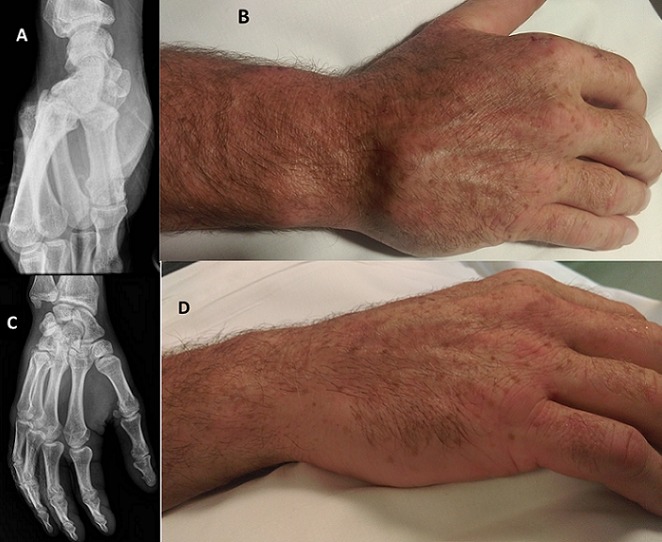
(A) radiographie standard de la main droite montrant la luxation carpo-métacarpienne du quatrième et cinquième métacarpiens; (B) la main droite montrant la luxation clinique carpo-métacarpienne du quatrième et cinquième métacarpiens; (C) radiographie standard de la main droite après réduction de la luxation carpo-métacarpienne du quatrième et cinquième métacarpiens; (D) la main droite après réduction
